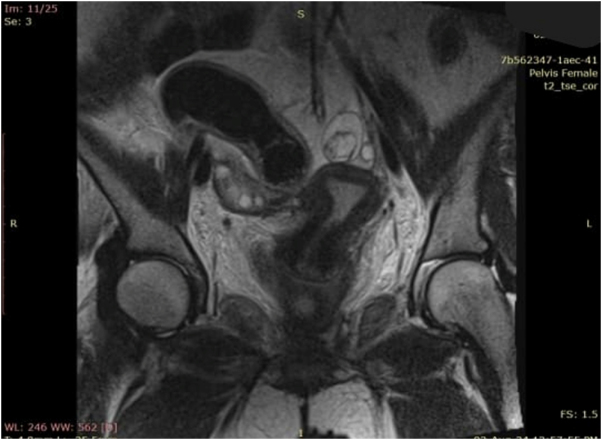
MRI pelvis showing left cystic adnexal mass consistent with an ovarian teratoma.
